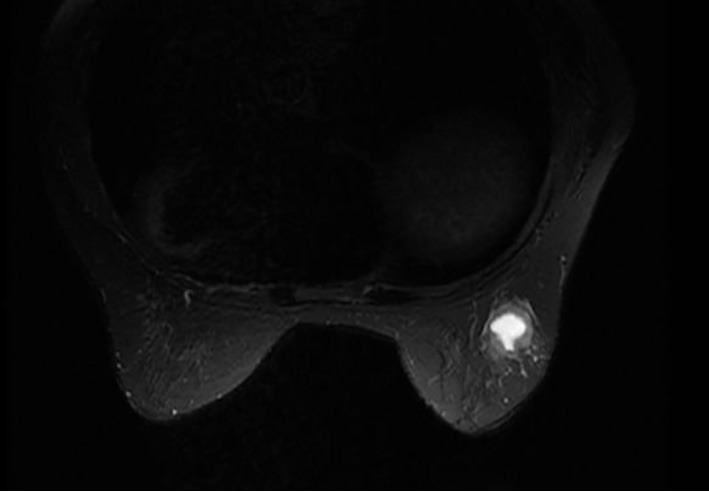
Preopetative breast MRI (T2 weighted image). MRI findings and a T2 weight image revealed an early enhancement image with no intraductal spread. MRI, magnetic resonance imaging.
